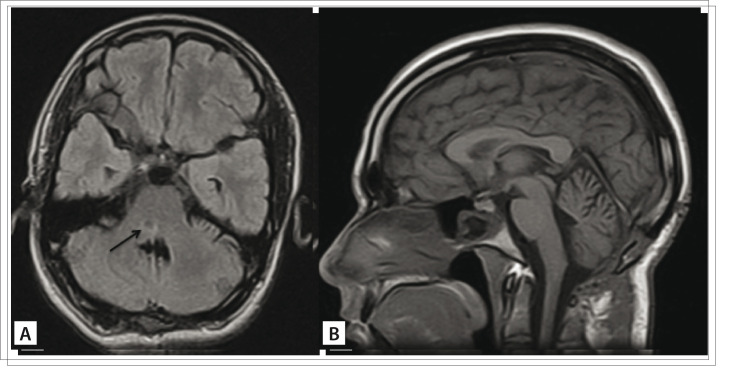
Magnetic resonance imaging produced in the sixth postoperative month, showing mild hypersignal within the pons (arrow), through FLAIR (fluid-attenuated inversion recovery) acquisition (A, axial view). No enhancement is seen with T1 acquisition (B, sagittal view)
